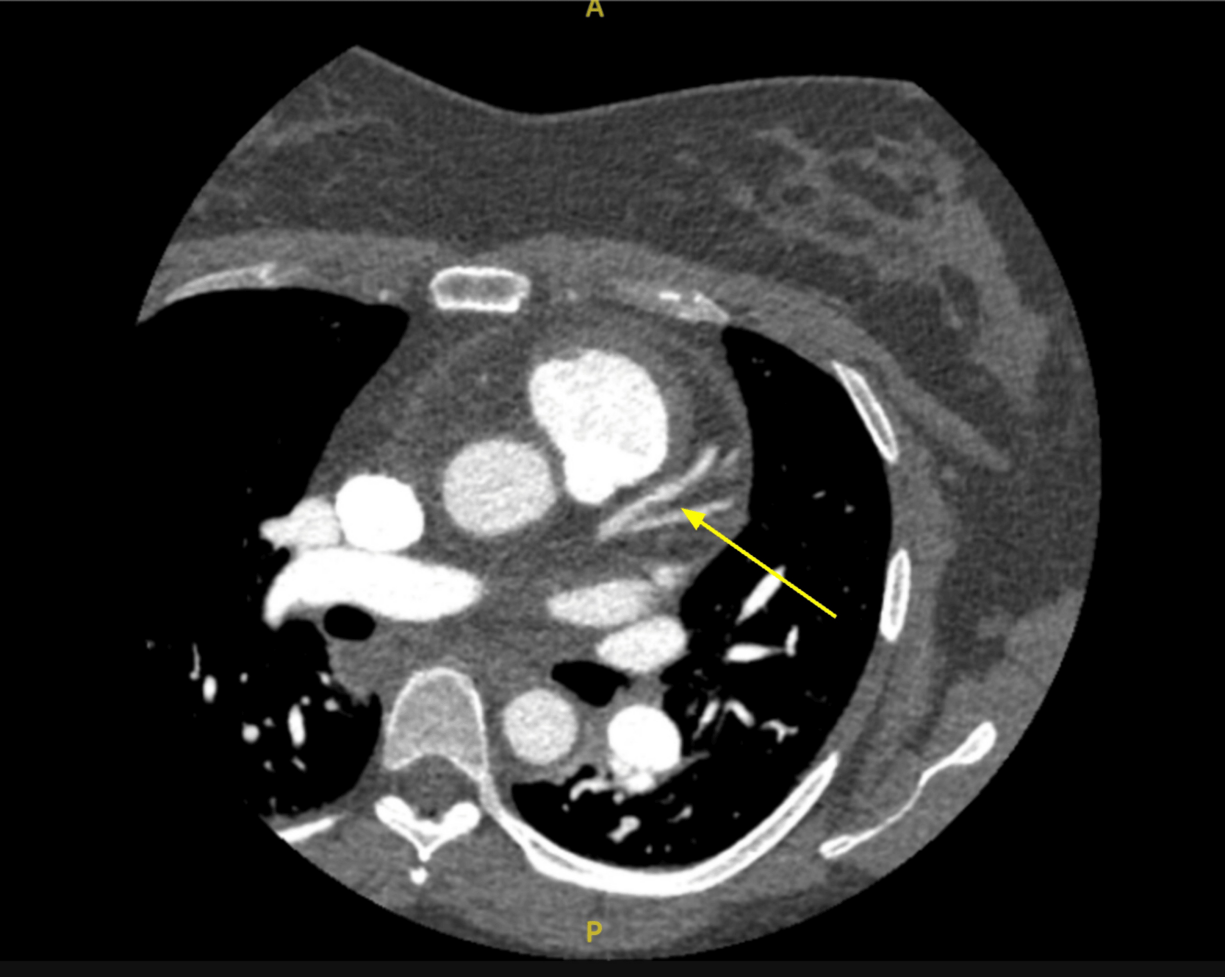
Computed tomography angiography. The yellow arrow points to the intramural hematoma form of spontaneous coronary artery dissection.
